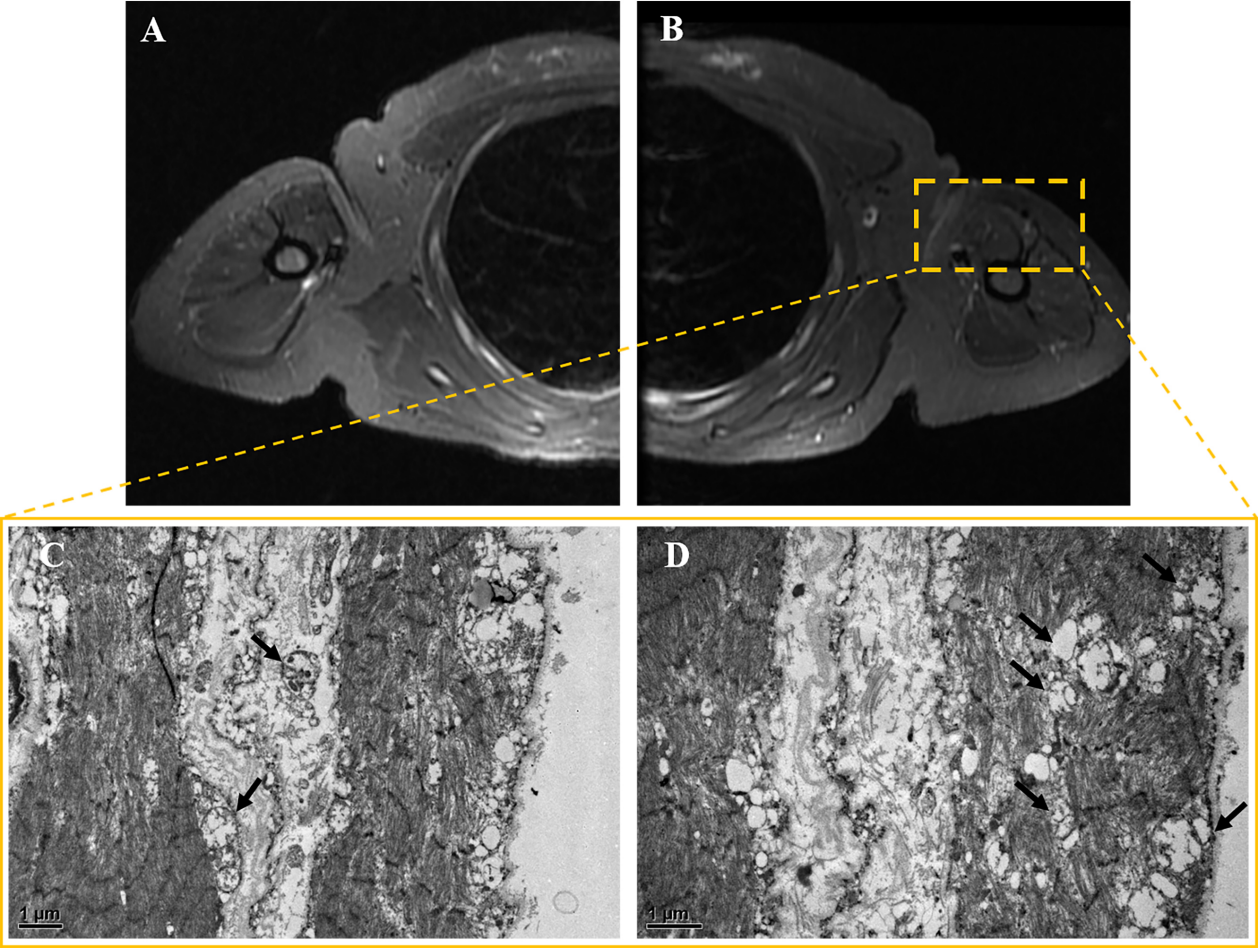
Muscle MRI and pathology results. (A,B) Bilateral
